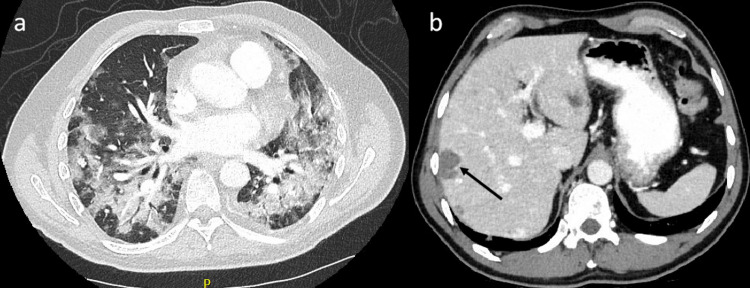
Repeat CT TAP images show progressive disease and superimposed pneumonia in the thorax (a), with increasing metastases in the liver (b) CT TAP: computed tomography of the thorax, abdomen, and pelvis
